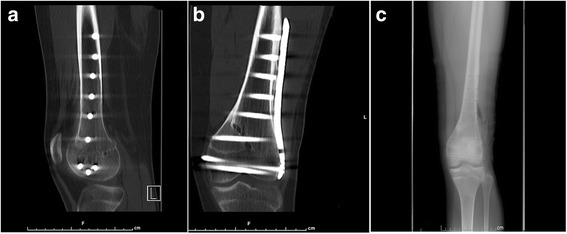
Six-month postoperative images of CT scans and X-rays. a, b Six-month postoperative CT scans anteroposterior and lateral views showing fracture consolidation. c Six months postoperative X-rays anteroposterior views of knee joint showing fracture consolidation after the removal of internal fixation
